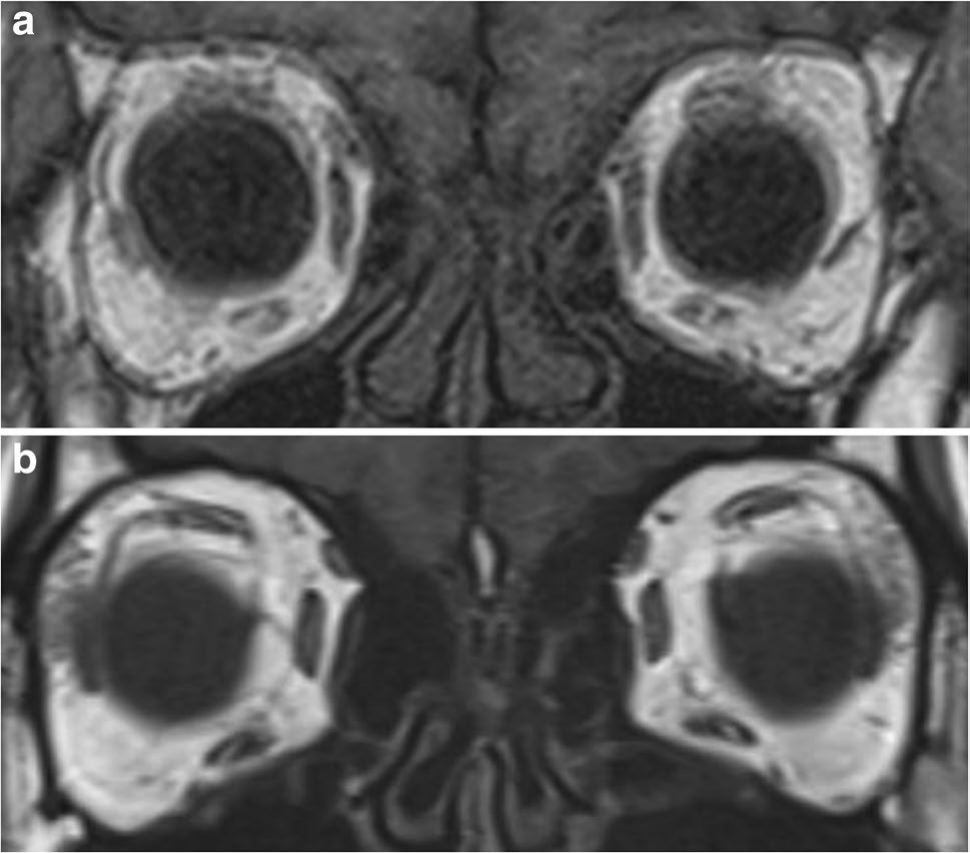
Orbital MRI. a A typical SES; MRI coronal section showed rupture of the LR-SR band and the temporally tilting of superior part of the LR only on the left eye. b A typical Trochlear nerve palsy; MRI coronal section did not show SO atrophy, LR-SR band rupture and LR inferior displacement
